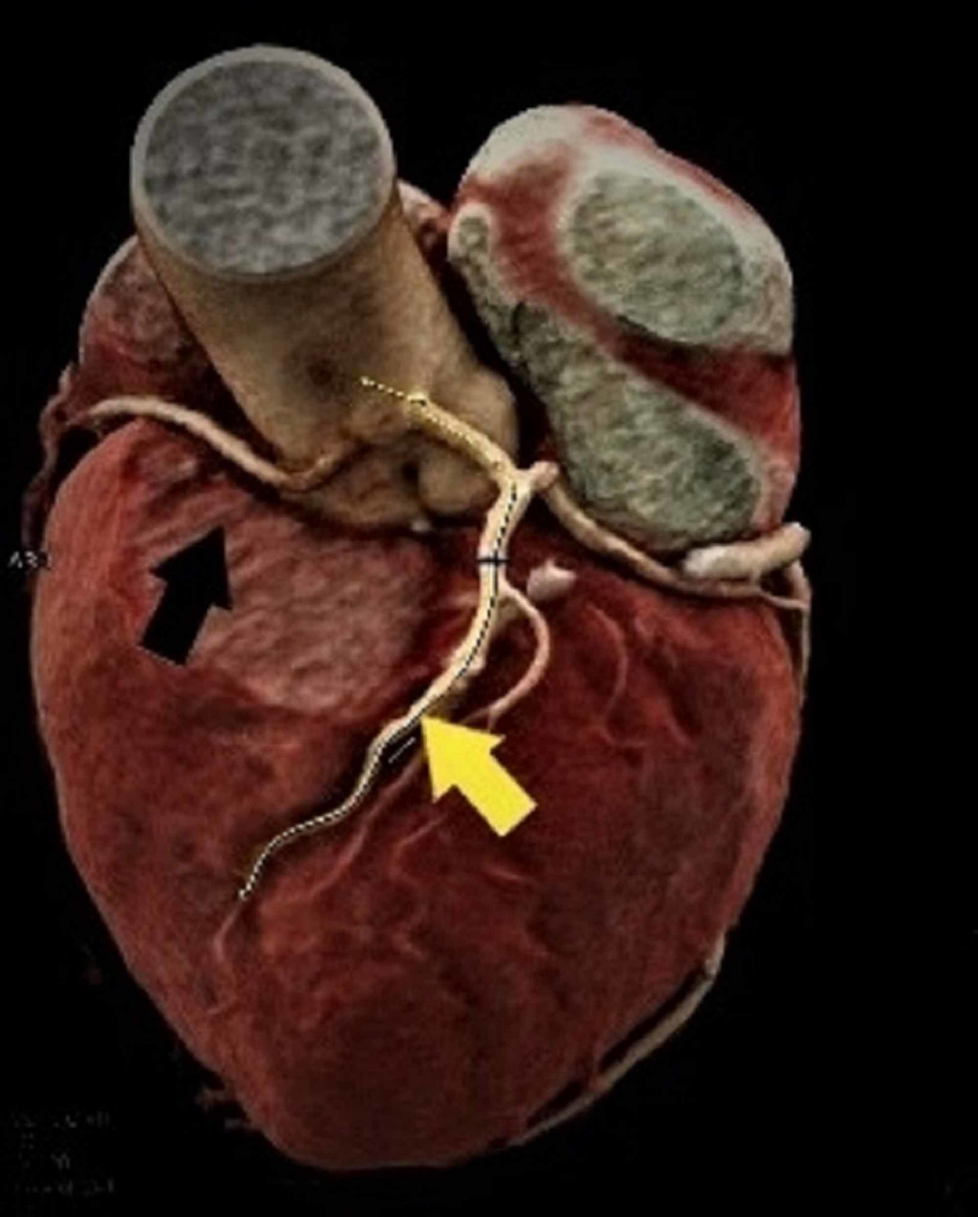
Three-dimensional CT imaging of the origin and course of the right coronary artery (black arrow) and the left coronary artery (yellow arrow)
